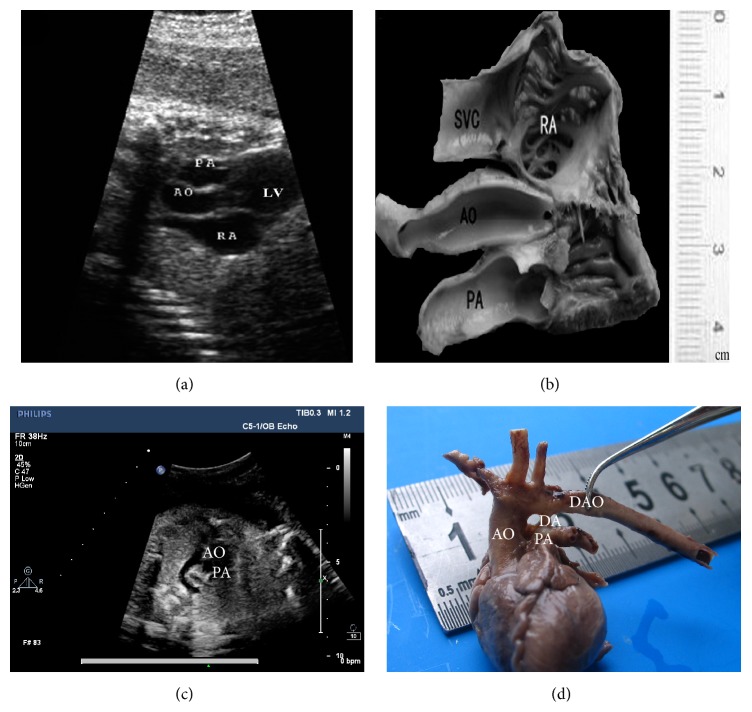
Aortic stenosis (AS) (38 w). (a) The superior mediastinum three-vessel echocardiography section. (b) The superior mediastinum three-vessel sectional anatomy. Pulmonary artery hypoplasia (PAH) (30 w). (c) The superior mediastinum three-vessel echocardiography section. (d) The superior mediastinum three-vessel sectional anatomy. SVC: superior vena cava; AO: Aorta; PA: Pulmonary Artery; RA: Right Atrium; LV: left ventricle; DAO: descending aorta; DA: ductus arteriosus.
